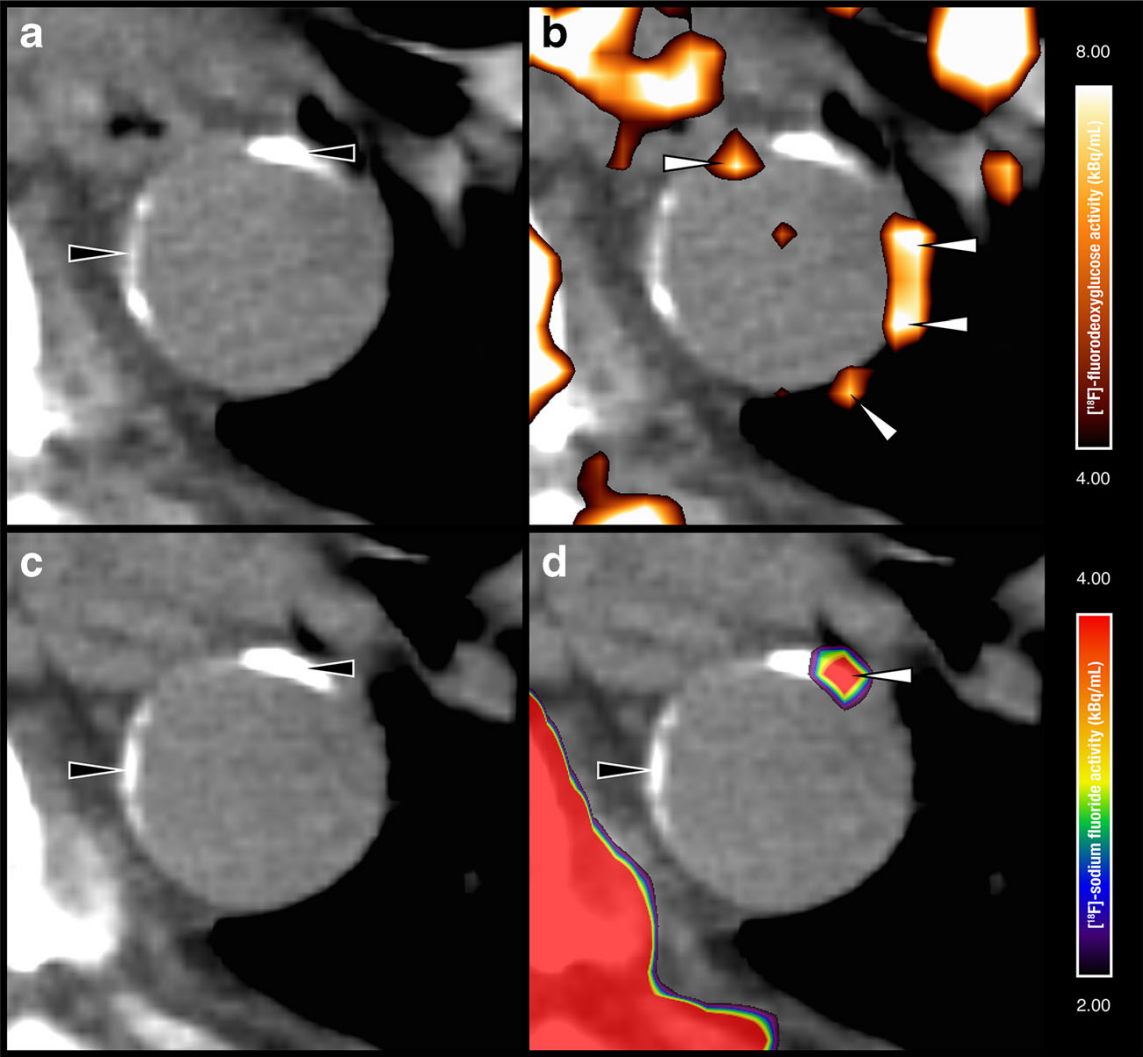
Axial CT (a, c), ^18^F-FDG PET/CT (b), and Na^18^F PET/CT (d) images obtained at the same location in 69-year-old man with hypertension, a body mass index of 28 kg/m^2^, and a Framingham risk score of 26 %. ^18^F-FDG accumulation is seen in the descending thoracic aorta (b
